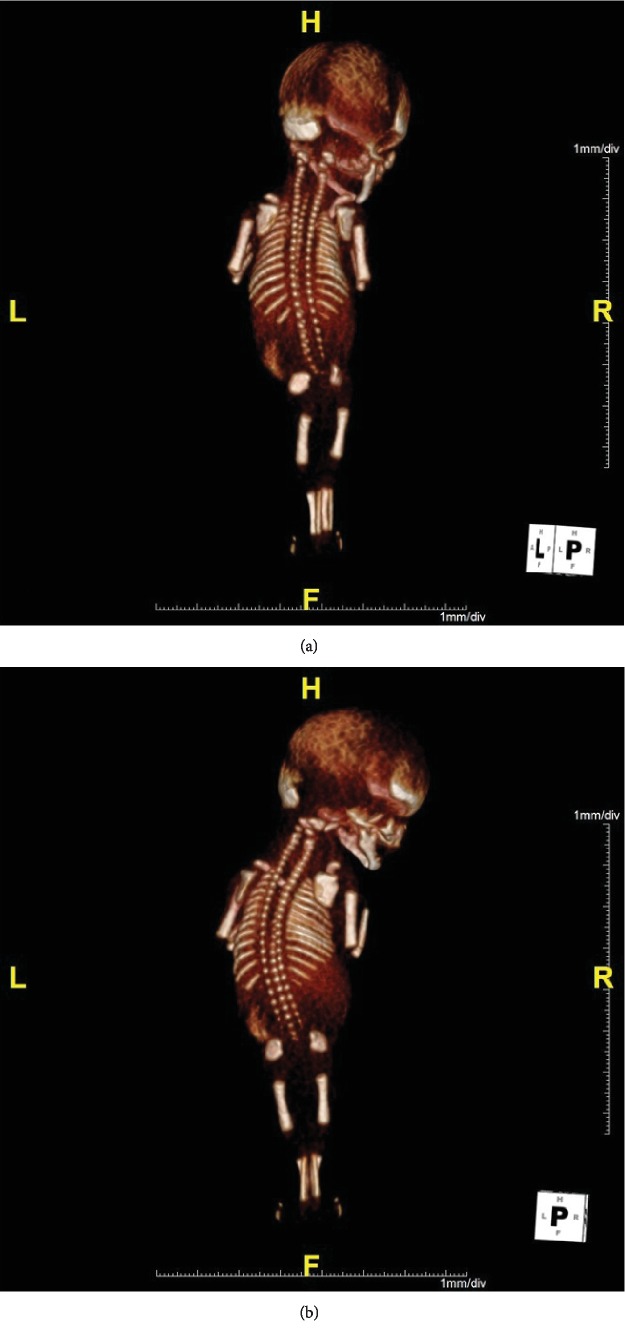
Postmortem CT images of the delivered baby. Two femurs, two tibiae, and two fibulae were contained in the single lower limb, and none of them was fused (a). Agenesis of lower lumbar and sacral spines was noted (b).
